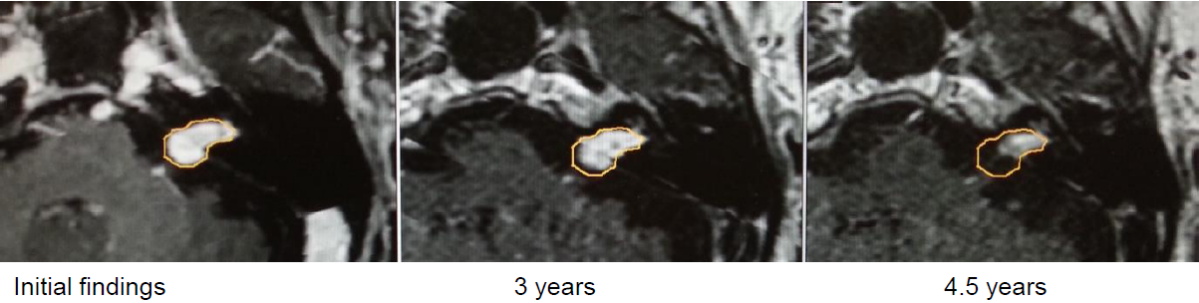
After 3 years, the MRI follow-up examinations (T1-w + CM) only show minimal size reduction, after 4.5 years, clear regression, the extrameatal part has nearly completely disappeared.
